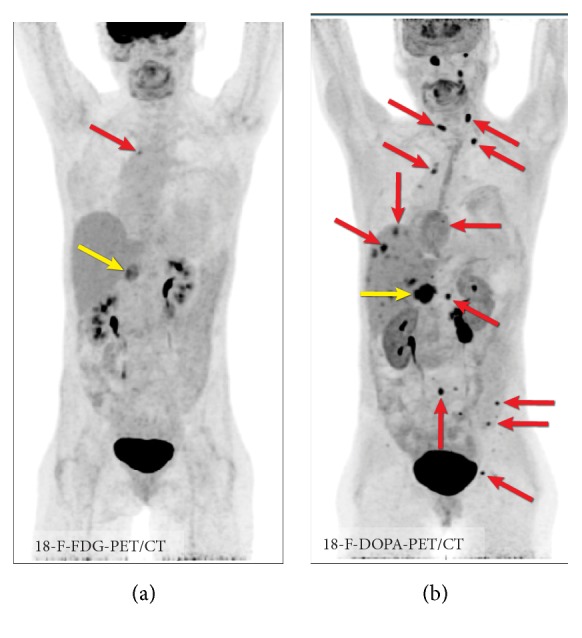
Imaging in MEN syndrome. 18F-FDG PET/CT (a) and 18F-FDOPA PET/CT (b) in MEN2B syndrome with metastatic pheochromocytoma (fractionated norepinephrine: 994 pg/ml, normal: 18-112 pg/ml; fractionated metanephrine: 1099 pg/ml, normal: 12-61 pg/ml) and metastatic medullary carcinoma (calcitonin: 5575, normal: < 8 pg/ml). In this case, the primary pheochromocytoma is seen in all images (yellow arrow); however, metastatic lesions are best visualized on 18F-FDOPA PET/CT (red arrows). Imaging cannot differentiate between medullary carcinoma metastases and metastases originating from pheochromocytoma.
